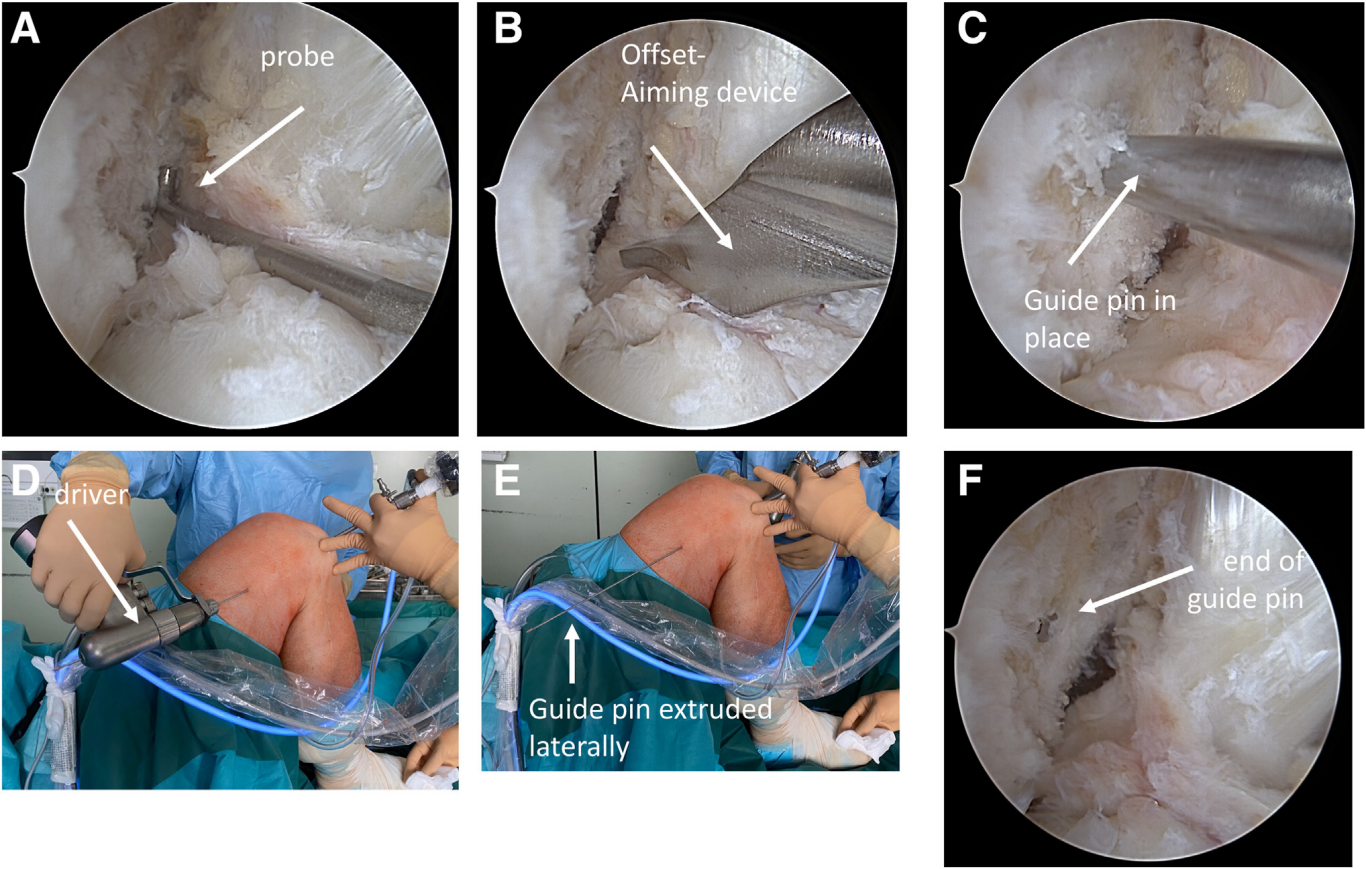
Technique of placing a guide pin into the lateral femoral condyle during anterior cruciate ligament (ACL) surgery and forwarding the pin so that a radiograph can be taken for analysis of its position. (A) Intercondylar view via anterolateral portal of a right knee after preparation of the lateral footprint with the probe at the dorsolateral aspect of the lateral femoral condyle. (B) Identical view with a 7-mm offset aiming device introduced through a low anteromedial portal in 125° of knee flexion. (C) Identical view with guide pin in place after removal of aiming device. (D) The guide pin is driven forward with the driver from the lateral aspect of the knee. (E) The tip of the guide pin can be seen at the lateral aspect of the knee. (F) The back end of the guide pin (red arrow) is flush with the cortex of the femoral condyle within the ACL footprint.
